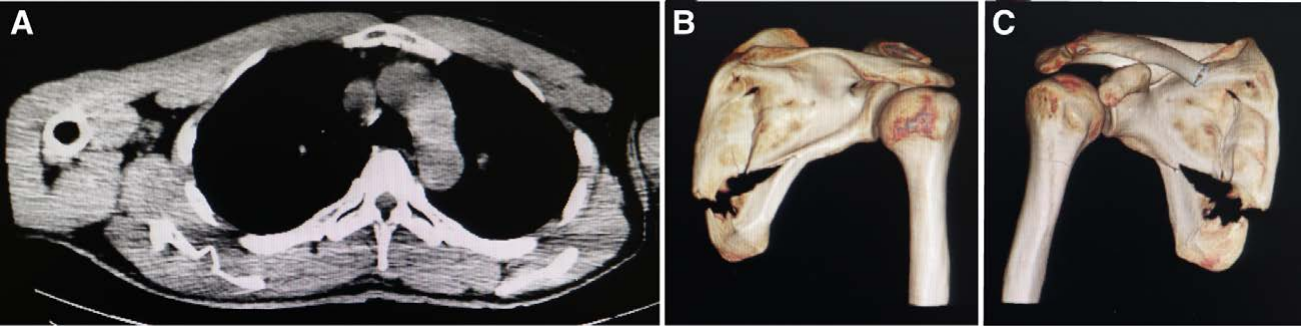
Partial examination results of case 1. (A) Chest CT scans showed a fracture of the right scapula without muscle tear or hematoma. (B, C) CT 3-dimensional reconstruction manifested multiple fractures of the right scapular body with displacement of the broken ends, no joints were damaged. CT = computed tomography.
